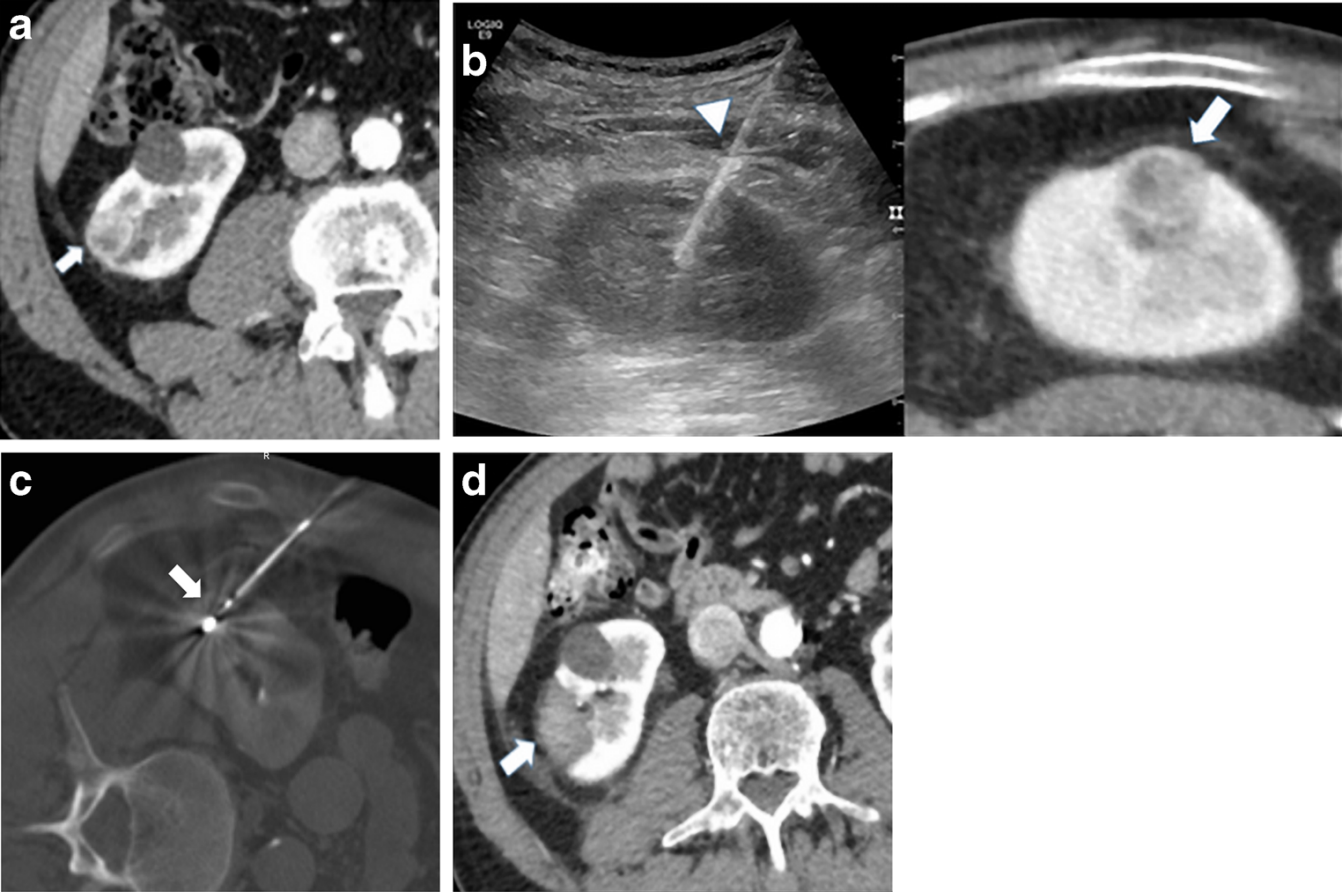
Treatment of a right renal tumour with the application of US/CT fusion imaging. a Contrast enhanced CT demonstrating a right renal tumour (*arrow*). b needle insertion was performed with fusion of real-time US with the preacquired CT that allowed the precise positioning of the microwave antenna (*arrowhead*) at the level of the tumour (*arrow*). c CT acquisition immediately after antenna insertion confirmed the correct positioning of the device (*arrow*) in the desired position. d contrast enhanced CT 24 h after treatment demonstrated the correct ablation of the tumour (*arrow*)
